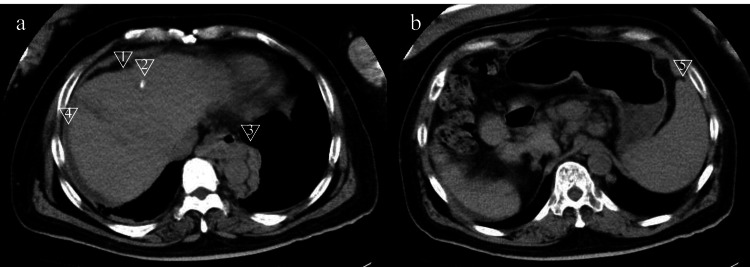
Computed tomography four months after admission. The images show (a) atrophic liver (arrow 1), a treated hepatocellular carcinoma nest (arrow 2), collateral veins (arrow 3), ascites (arrow 4); and (b) splenomegaly (arrow 5), which remained unchanged during hospitalization.
